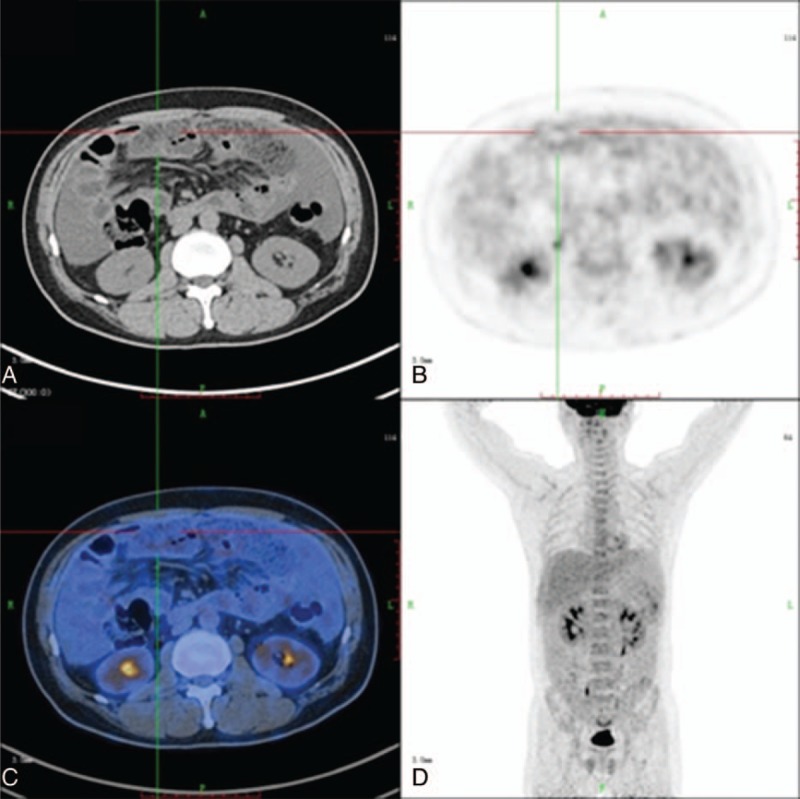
Images of 51-year-old man who presented with abdominal pain for 3 months: axial CT (A), axial PET (B), axial fused PET/CT (C), and 3D PET (D). The patient had serum CA12-5 of 355 U/mL. Noninvasive examinations could not detect the primary cause of the peritoneal thickening. PET/CT images show normal uptake in the gastric area (SUV_max_ of 1.5). However, gastroscopy was repeated, and biopsy confirmed a malignant gastric lesion. CT = computed tomography, PET = positron emission tomography, SUV_max_ = maximal standardized uptake value.
